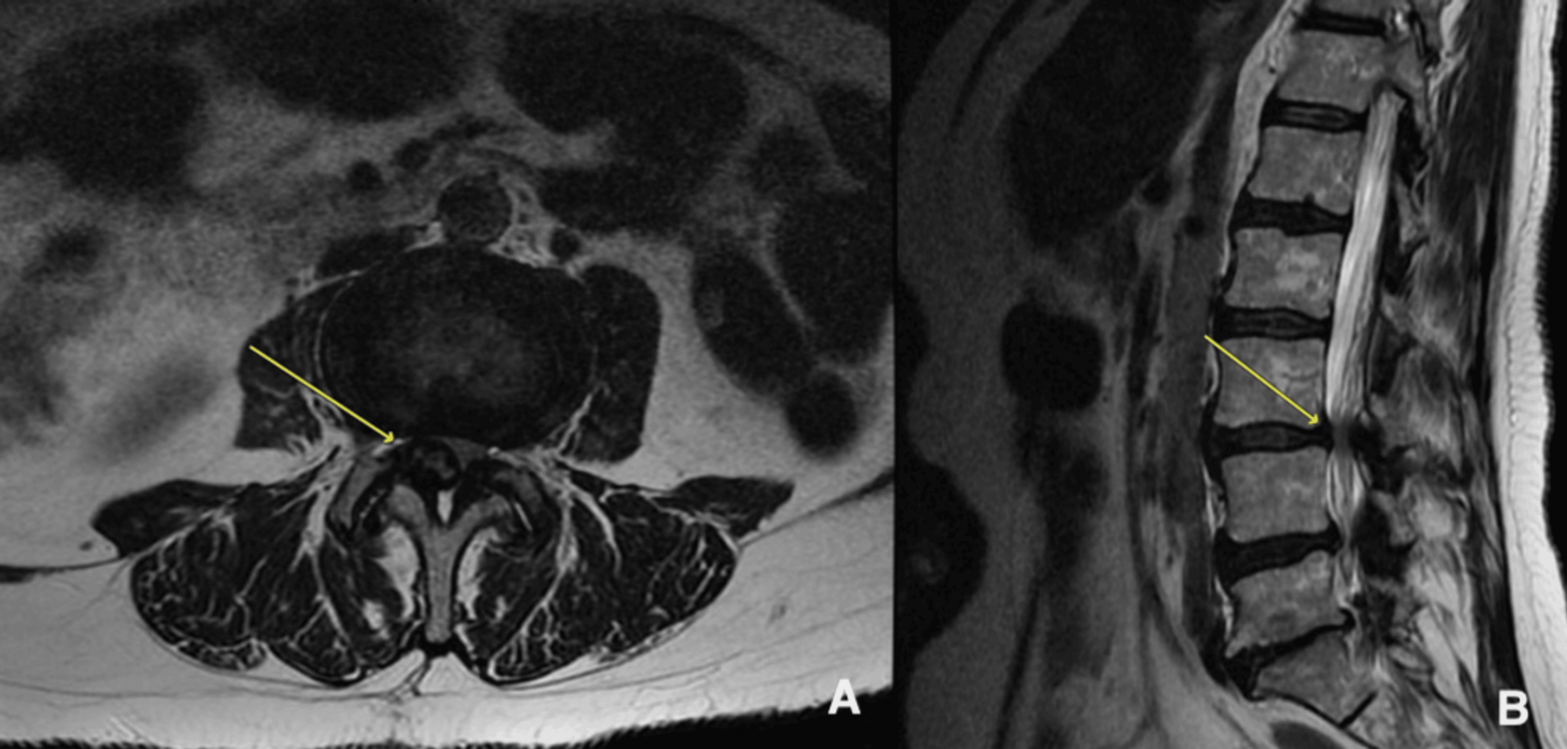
MRI of the lumbar spine demonstrating a facet cyst at L3-L4 (yellow arrows). (A) axial view; (B) sagittal view
